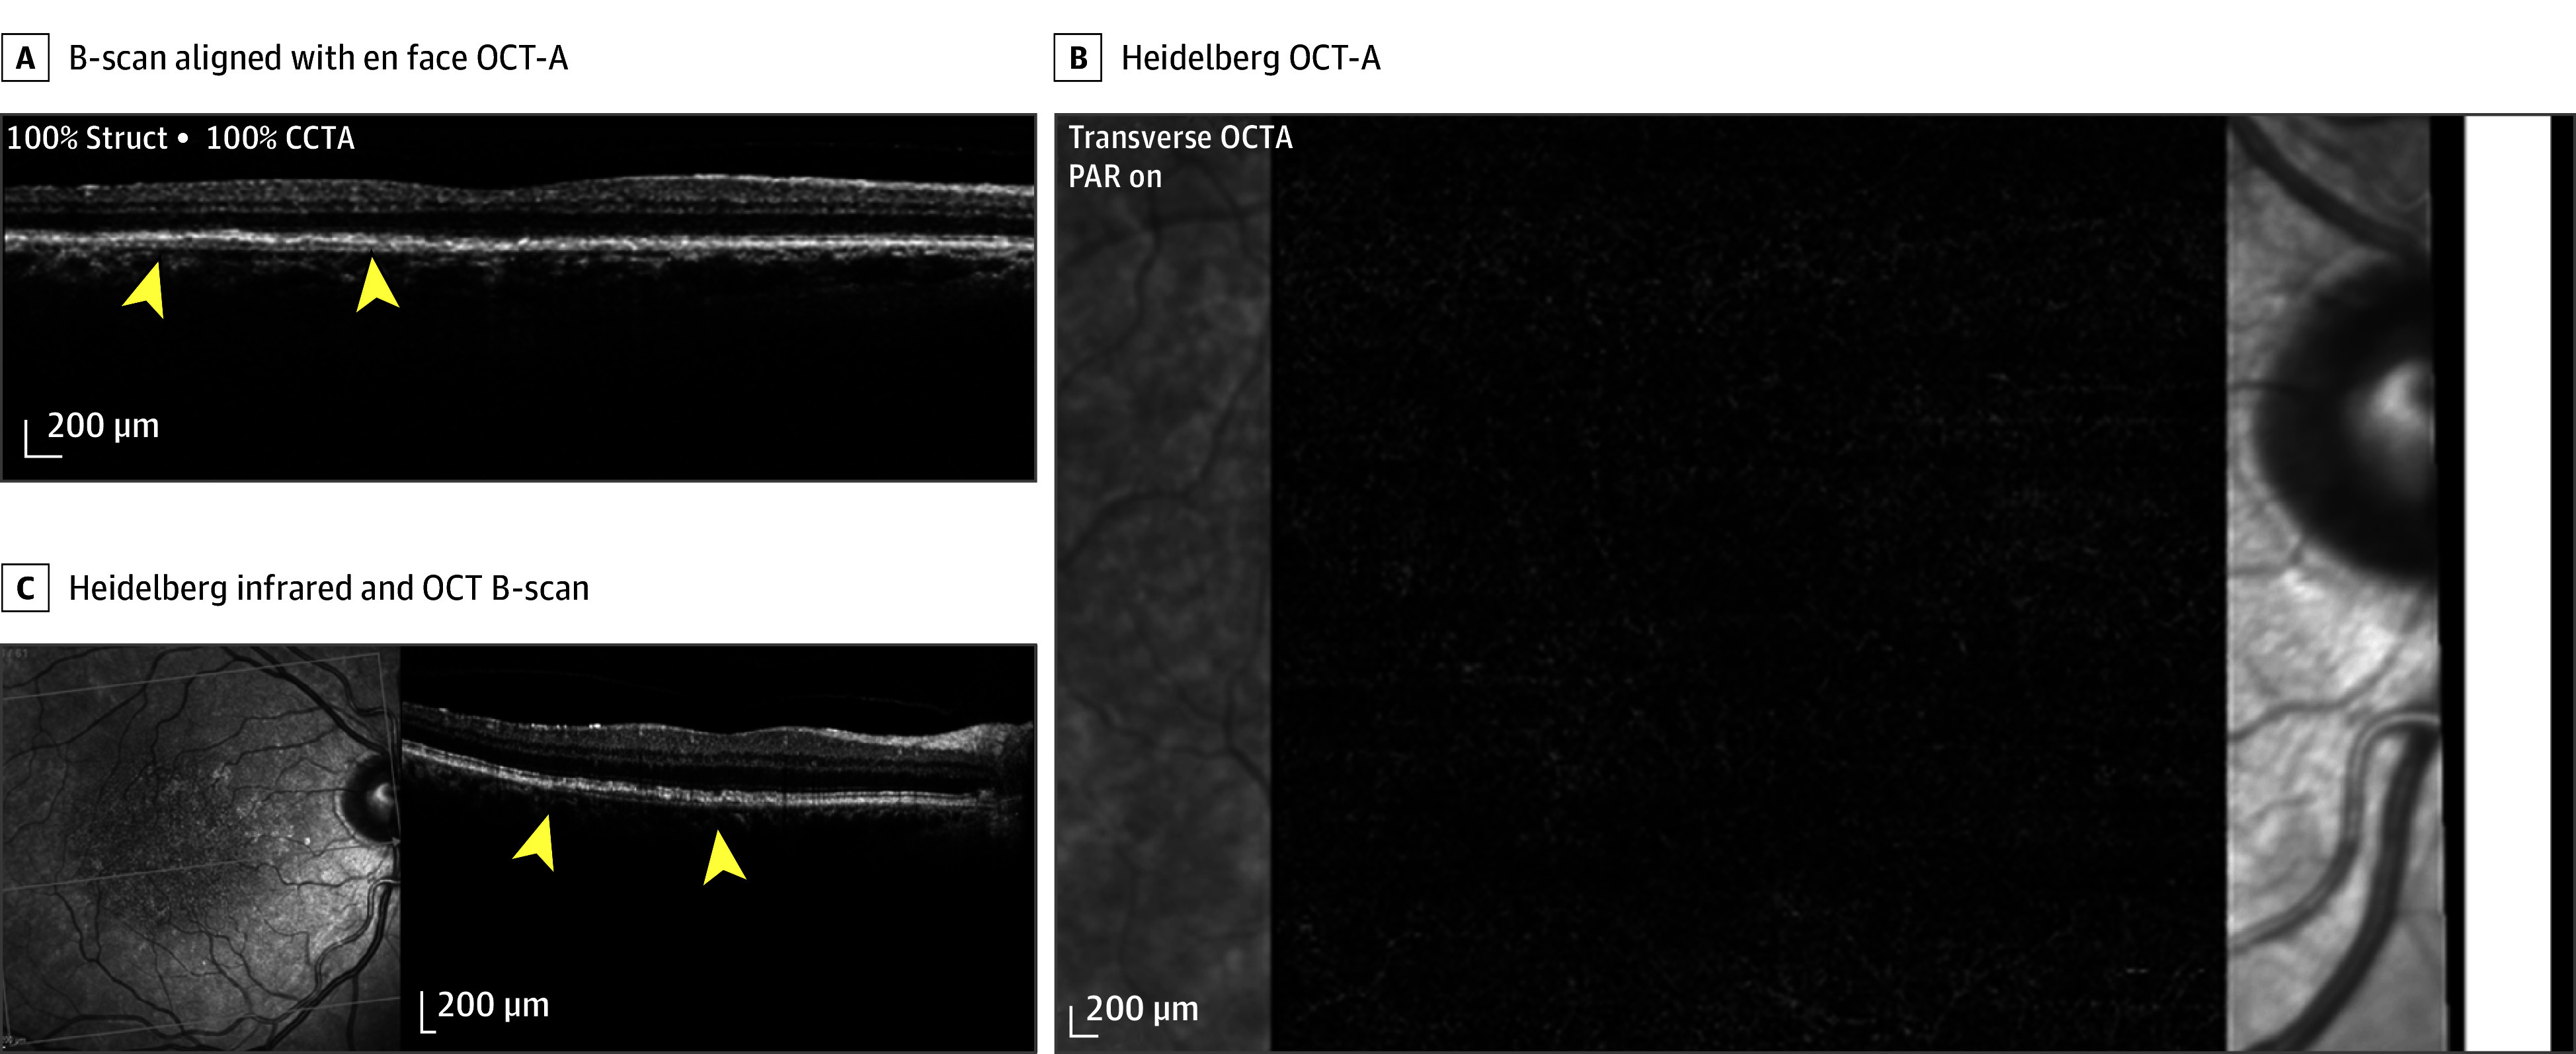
Imaging Showing a Thin Double-Layer Sign That Does Not Contain a Nonexudative Neovascular Membrane A, The brightness scan (B-scan) that aligns with the enface optical coherence tomography angiography (OCT-A) image and the boundary is indicated by yellow arrowheads. B, Heidelberg OCT-A showing the absence of nonexudative neovascular membrane. C, Heidelberg infrared and OCT B-scan showing the double-layer sign and the boundary is shown indicated by yellow arrowheads. CCTA indicates coronary computed tomography angiography; PAR, persistent avascular retina.
